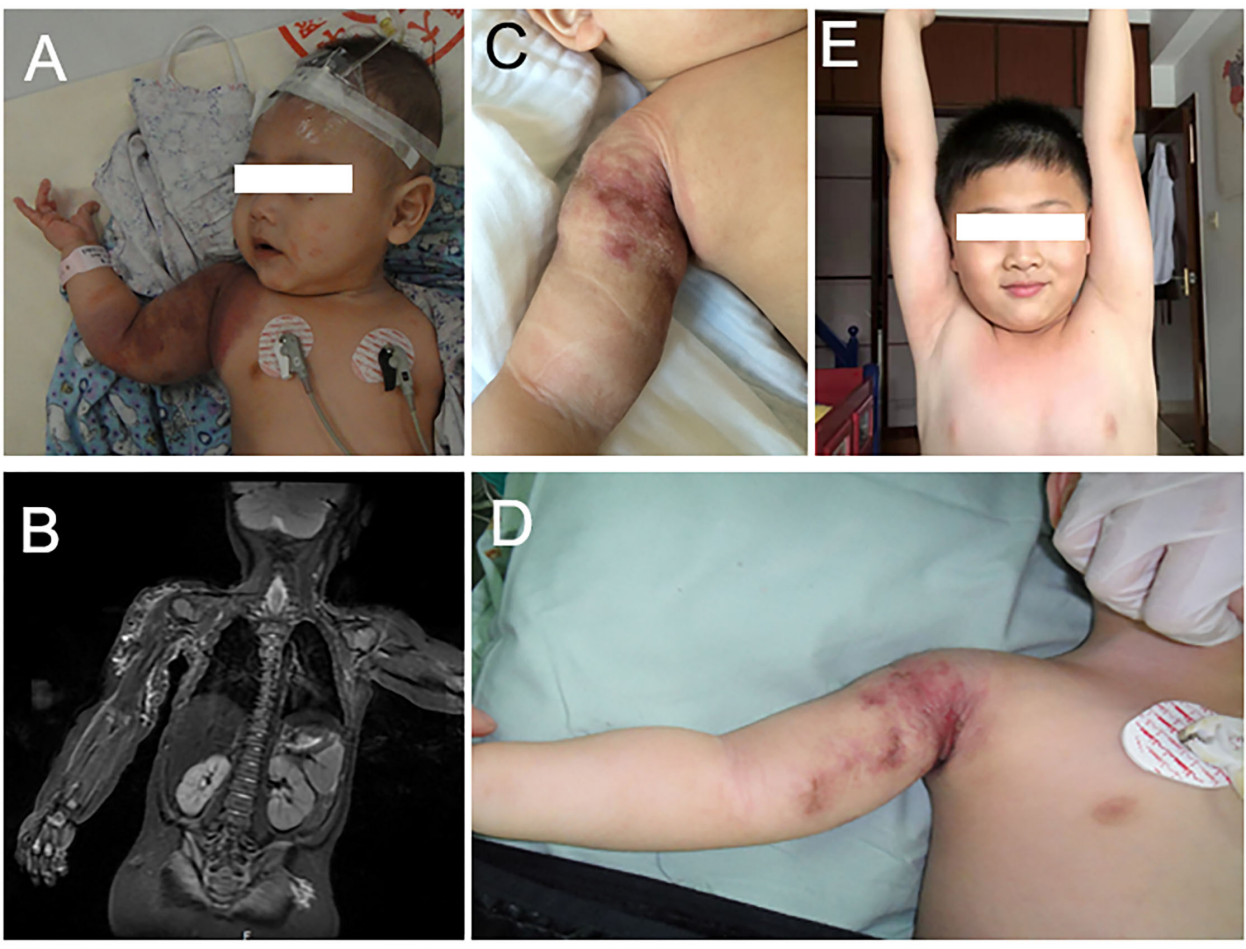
(A) The patient was diagnosed with KHE with KMP on his right shoulder and arm at 4 months. (B) Magnetic resonance imaging with contrast enhancement revealed the vascular lesion on his right shoulder and arm. (C) The lesion recessed obviously after three courses of ILP and sclerotherapy. (D) The tumor recessed after three courses of ILP and sclerotherapy. (E) The tumor absolutely recessed at 6 years.
